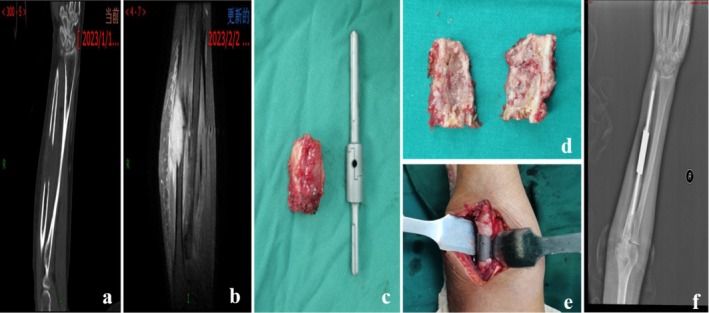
A diaphyseal mortise‐tenon prosthesis was implanted in patient 2 for a metastatic lesion of the right ulnar diaphysis. Preoperative (a) computed tomography scan and (b) MR image show metastatic lesion of the right ulnar diaphysis. (c and d) The resected tumor segment and the customized diaphyseal mortise‐tenon prosthesis are shown. (e) Intraoperative photograph shows implantation of a diaphyseal mortise‐tenon prosthesis. (f) Postoperative X‐ray image shows that the prosthesis is in good position.
